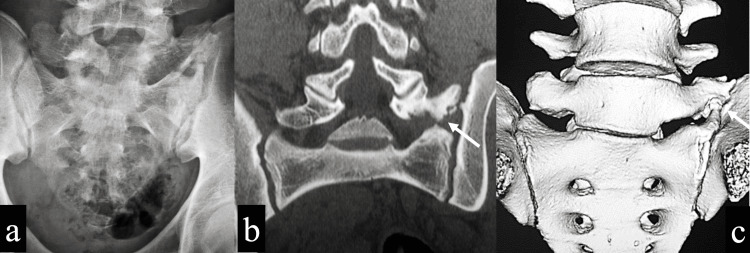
One-year postoperative imaging findings a: Plain X-ray anteroposterior view showed bony regeneration around the transverse process. b: CT coronal view showed bone regeneration of the transverse process and sacral alar (white arrow). c: CT 3D image showed no bony continuity of the transverse process and sacral alar or iliac crest (white arrow).
